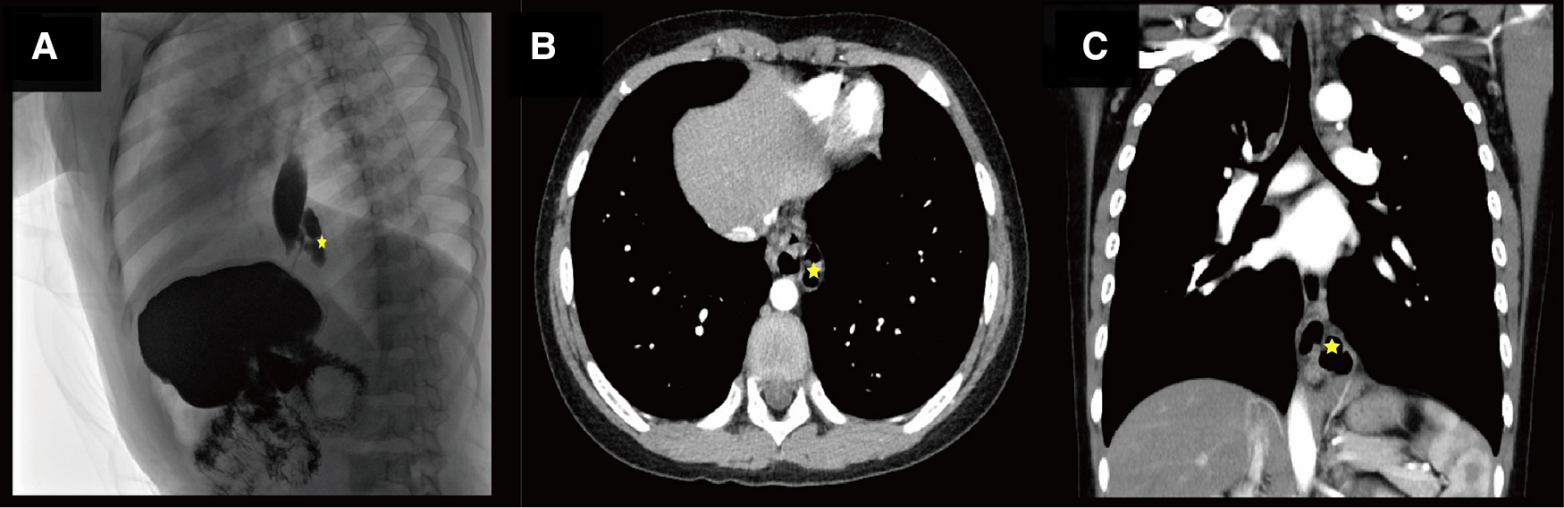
(A) Shows the radiological investigation of the child. Star indicates an irregular lumen shadow behind the lower esophageal segment on the lateral view of the upper gastrointestinal contrast study before the operation. (B) Shows the radiological investigation of the child. The star indicates an irregular lumen in the left posterior mediastinum of the contrast-enhanced computed tomography (CT) before the operation. (C) Shows the radiological investigation of the child. The star shows an irregular lumen shadow adjacent to the lower esophagus on coronal contrast-enhanced CT before the operation.
